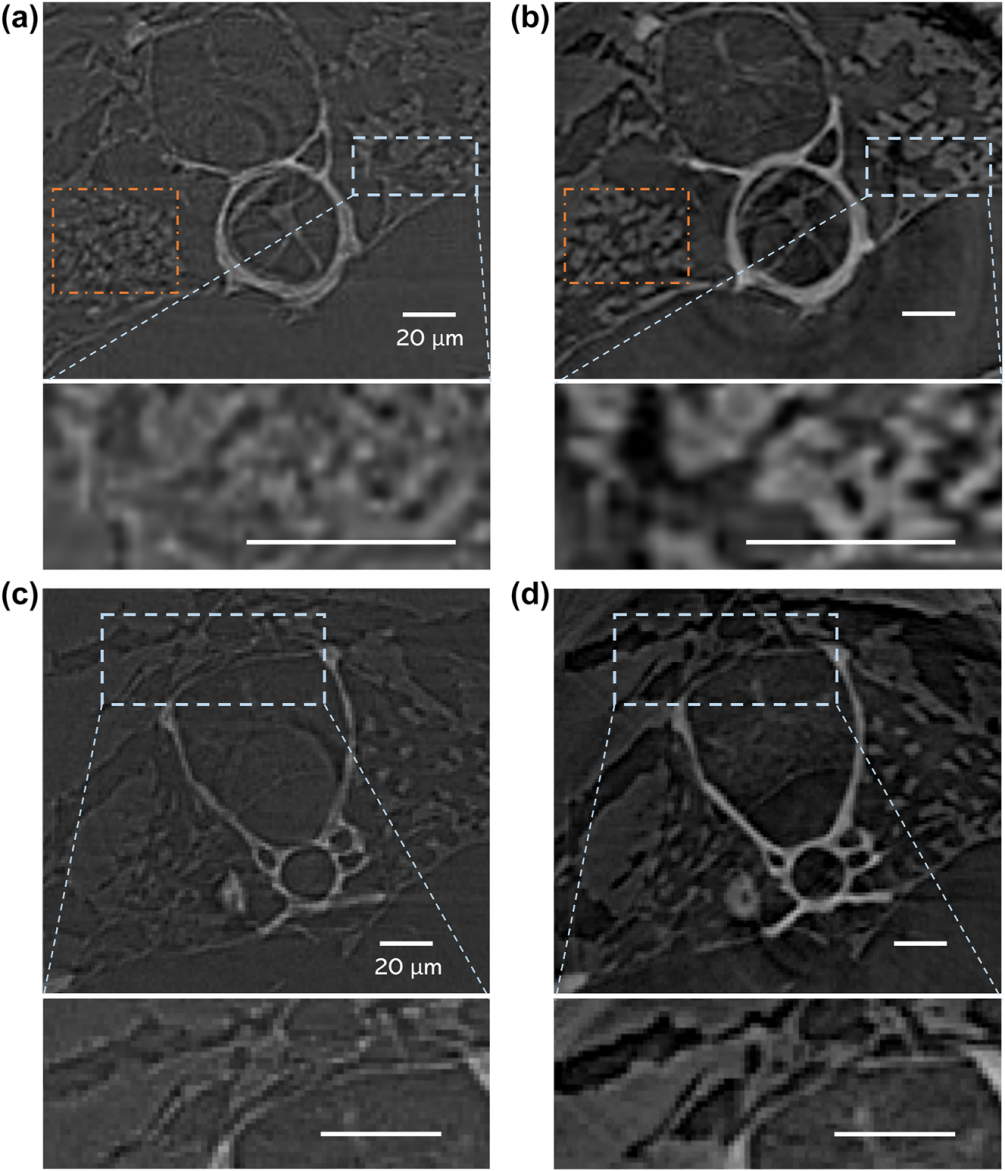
Tomography results for different X-ray imaging frameworks. Reconstructed slices and the enlarged view of the select area of the vertebra via (a) *Nsys* and (b) *SysK*
